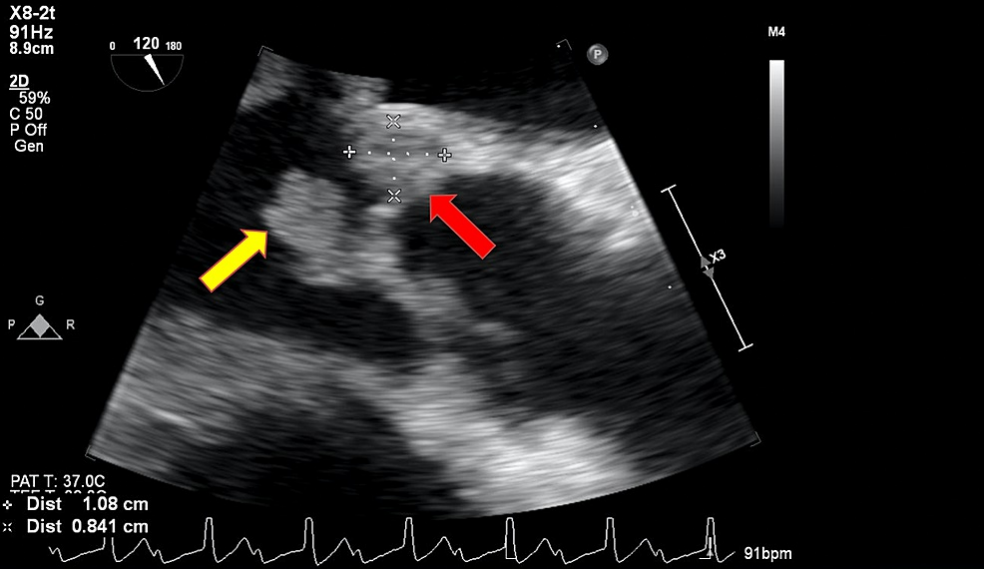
Transesophageal echocardiogram showing a large aortic root abscess (red arrow) and aortic valve vegetation (yellow arrow)
